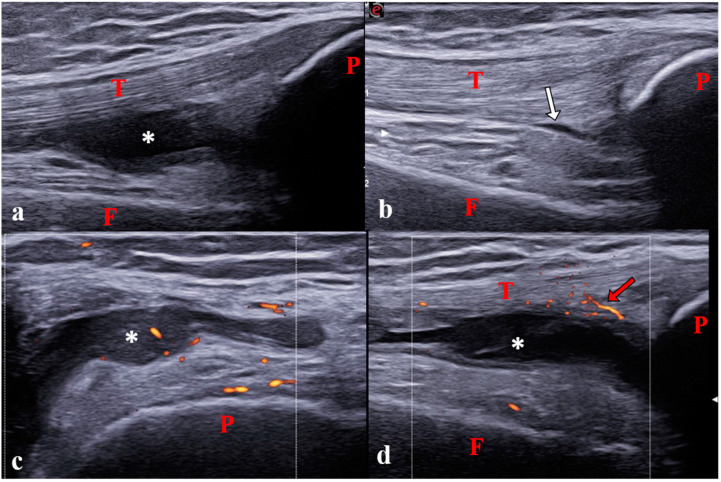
Synovitis of the knee joint in a 12-year-old girl with polyarticular juvenile idiopathic arthritis. (a) Longitudinal suprapatellar scan showing synovitis in the suprapatellar recess on B-mode (grade 2). (b) Physiological aspect of the contralateral joint on B-mode. (c) Transverse lateral parapatellar scan showing pathological vascularization (power Doppler grade 1). (d) Longitudinal suprapatellar scan showing vascularization outside the synovial hypertrophy (power Doppler grade 0). Note that the transverse scan is more sensitive for detecting pathological vascularisation inside synovitis than the longitudinal scan [18]. P: patella; T: tendon; F: femur; *: Synovitis; white arrow: small physiological amount of intra-articular fluid; Red arrows: physiological vascularization.
